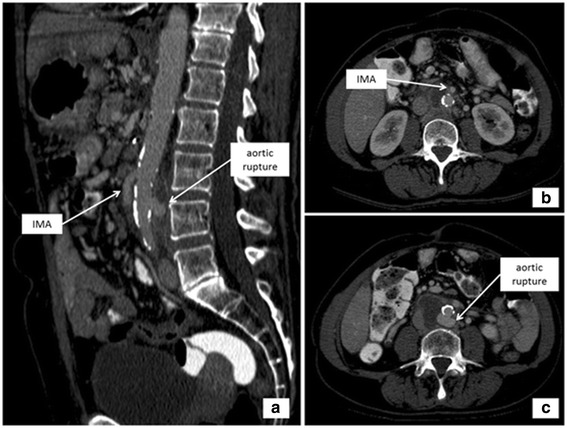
Sagittal reconstruction of computed tomography scan (a) and axial images (b, c) revealed abdominal aortic pseudoaneurysm at the dorsal circumference of the aorta below the ostium of the IMA due to tumor relapse
